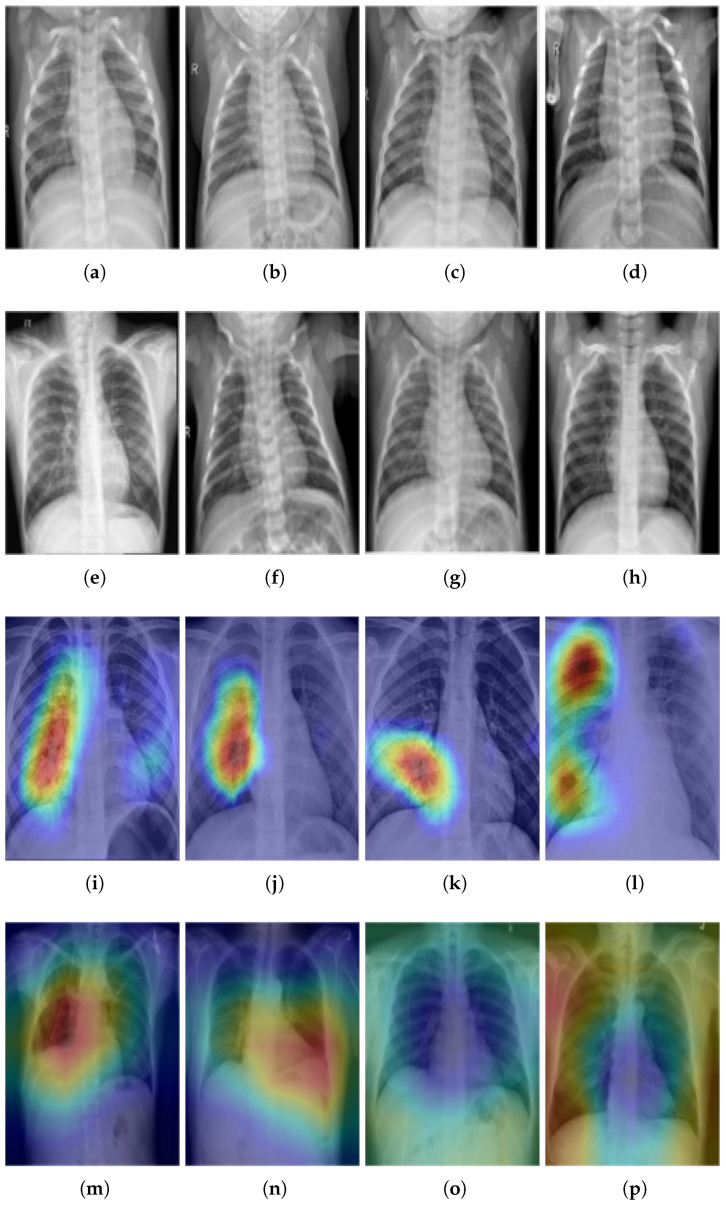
(a–h) X-ray images of normal patients, (i–p) X-ray images of pneumonia patients.
